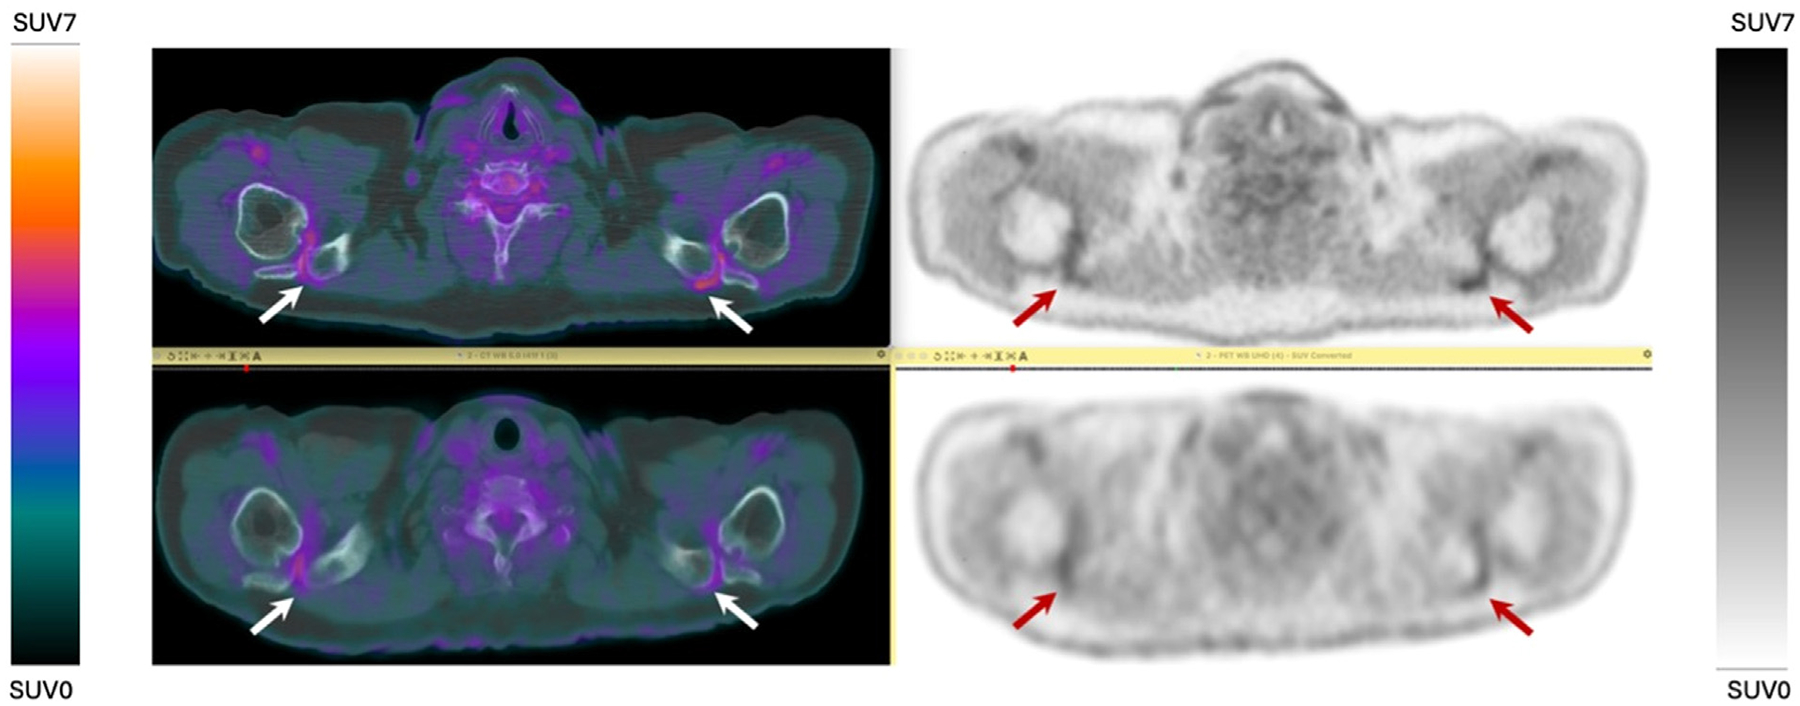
PET/CT of a 72-year-old patient with lung cancer, presented for evaluation of distant metastasis. Axial view images from total-body PET (top) and SAFOV scanner (bottom) were acquired on the same day after an IV injection of 368 MBq [^18^F]FDG. Radiotracer activity is noted at the bilateral acromioclavicular joints. Images obtained on LAFOV scanner demonstrate better spatial resolution and signal-to-noise.
